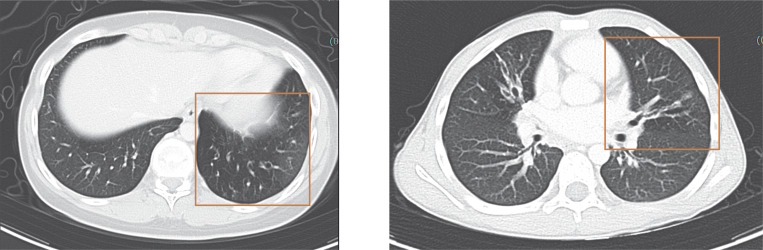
Chest CT. Representative axial chest CT images showing bronchiectasis. Bronchiectasis lesions are highlighted in orange boxes. Left and right panels depict patients aged 14 and 10 years, respectively.
